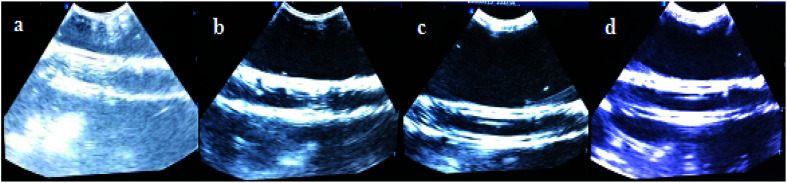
Ultrasound images of the different groups *in vitro* for: (a) normal saline, (b) Span–PEG microbubbles, (c) Span–PEG with CNT microbubbles, (d) Span–PEG with FA–CNT–PTX microbubbles.
